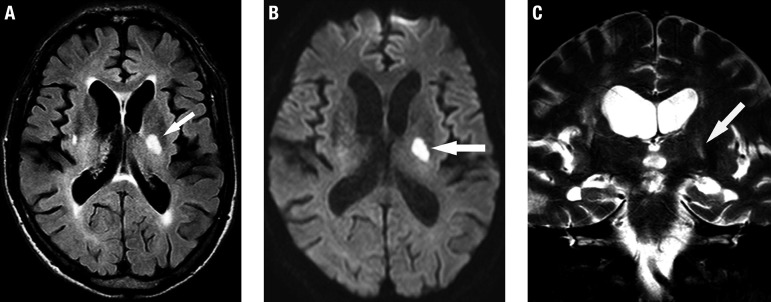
Recent small subcortical infarct. A 75-year-old man with sudden onset of left hemiparesis. Axial FLAIR (A), diffusion-weighted (B) and coronal T2-weighted (C) images demonstrate a recent small infarct in the posterior limb of the left internal capsule (arrows). Note the concomitance of white matter hyperintensities of presumed vascular origin on FLAIR (A) and T2-weighted (C) images. Diffusion-hyperintensity, indicating restriction (arrow in B) corroborates that this represents a recent lesion, and is correlated with the sudden clinical onset. On coronal T2-weighted (C) image, the elongated morphology of the infarction in the superoinferior axis is evident, related to the territory of a perforating artery.
